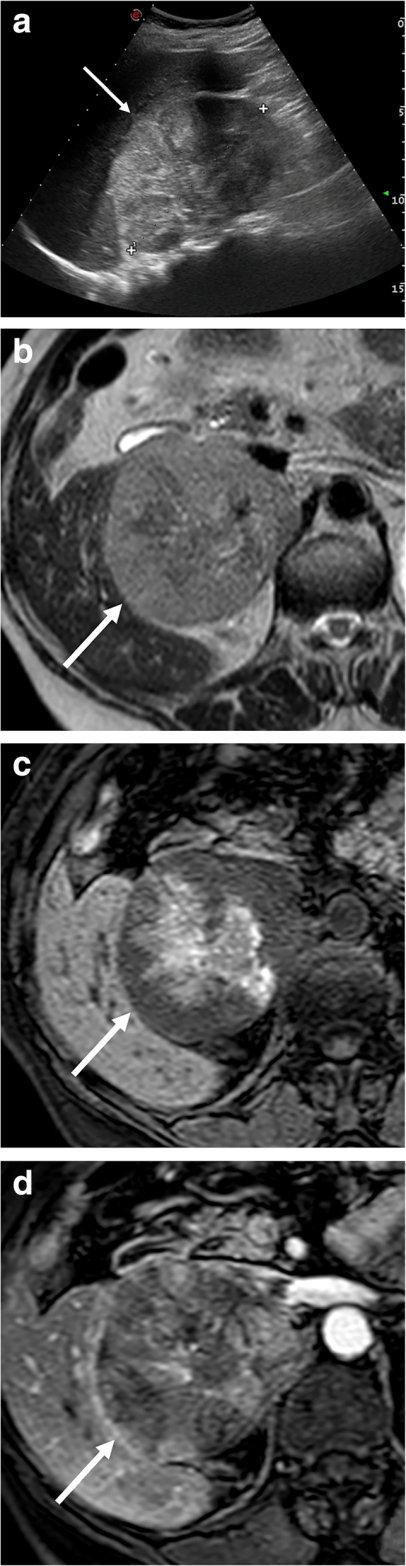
Right cortical carcinoma in a 70-year-old woman admitted to the emergency department for atraumatic abdominal pain. US (a) shows a large heterogeneous right adrenal mass (arrow). Axial T2-weighted spin echo (b), three-dimensional fat-suppressed T1-weighted gradient recalled echo images before contrast injection (c), in arterial phase (d) images confirm the presence of a large right adrenal mass with necrotic and hemorrhagic components which present as areas of high signal on unenhanced T1-weighted (c) images and non-enhancing areas after contrast injection (d)
